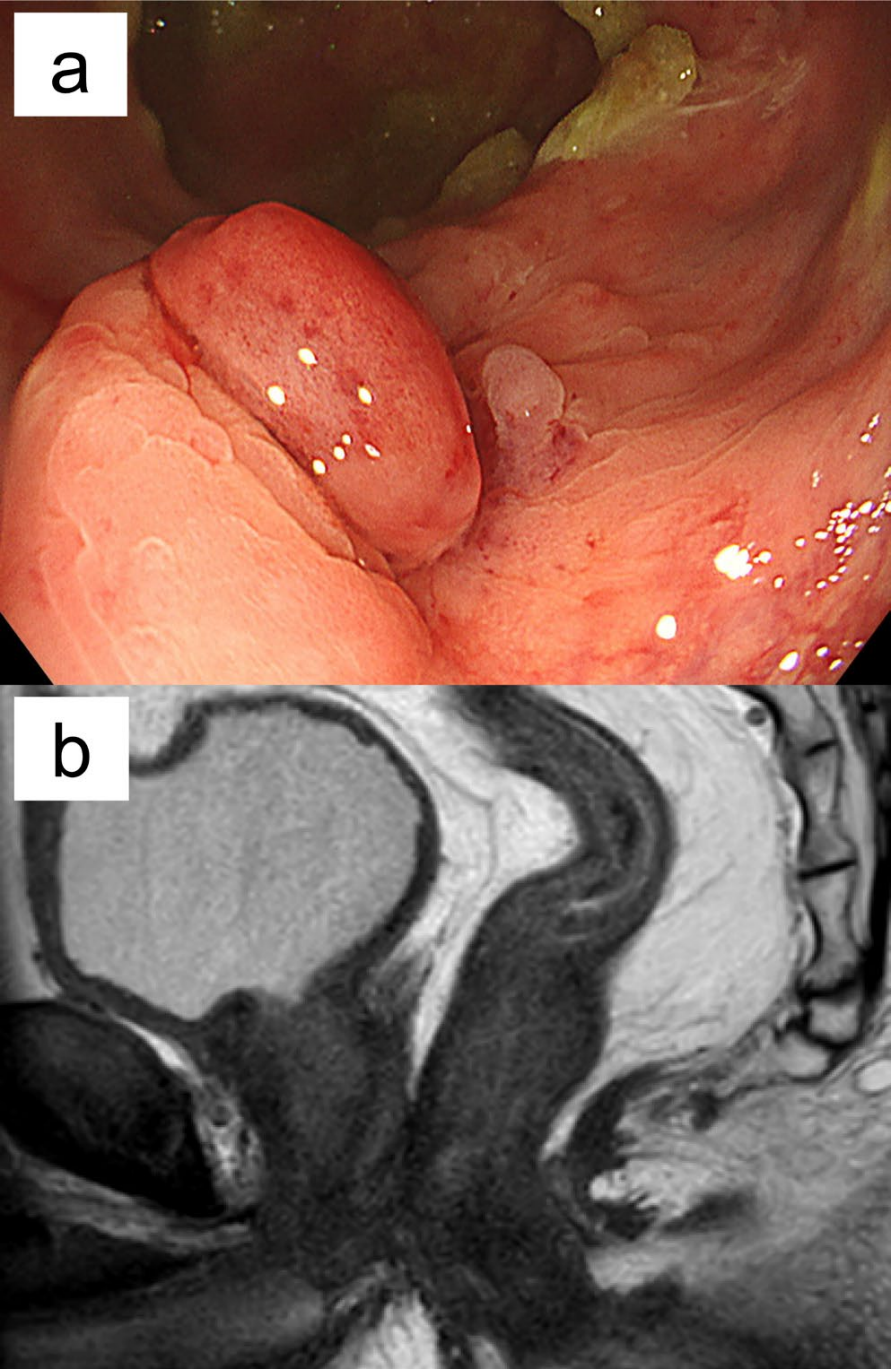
(a) Colonoscopy showing granulation tissue formation. No surrounding fistula or mucosal defect; scarring is present. (b) Sagittal T2‐weighted MRI showing resolution of previously observed low signal in the anterior wall.
